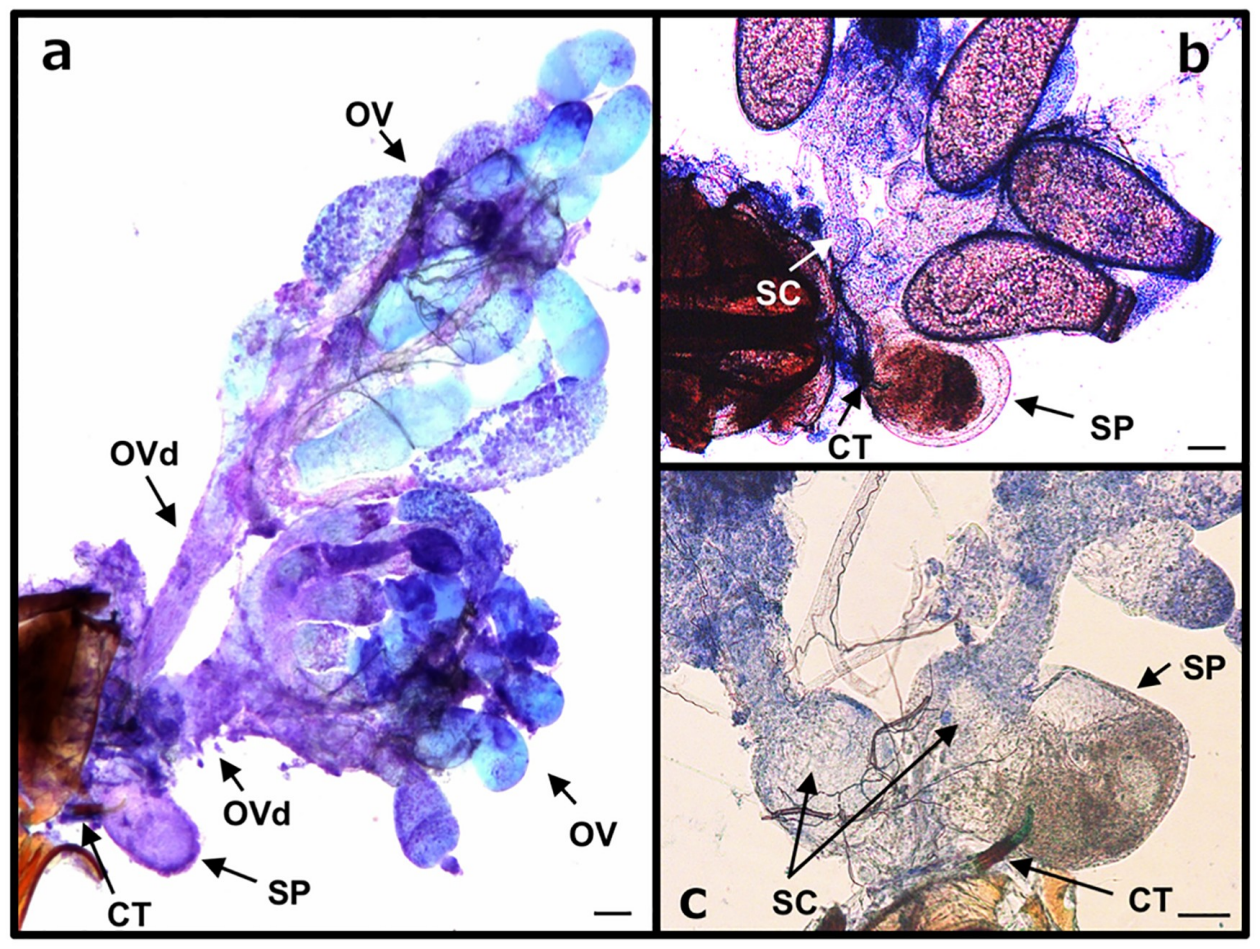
Reproductive organs of *O*. *strigicollis*. The ovary from a one-week-old virgin female (a), two-week-old mated female (b), and an approximately three-week-old mated female (c). The seminal conceptacles were hidden by the integument in Panel a. OV, ovary; OVd, oviduct; CT, copulatory tube; SP, sperm pouch; SC, seminal conceptacles. Scale bars in each panel indicate 100 μm.
